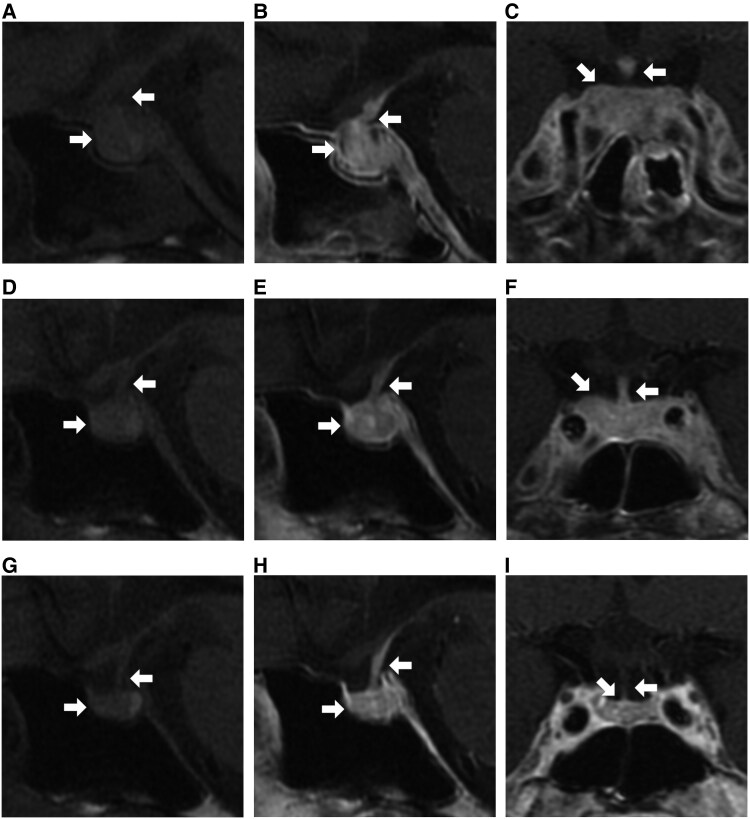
Serial pituitary magnetic resonance imaging (MRI). Noncontrast-enhanced T1-weighted sagittal images (A, D, and G), contrast-enhanced T1-weighted sagittal images (B, E, and H), and contrast-enhanced T1-weighted coronal images (C, F, and I) are shown. On admission (A–C), diffuse enlargement and homogeneous contrast enhancement of the pituitary gland and stalk (white arrow) were observed without optic chiasm compression. On day 21 (D–F), reduction in pituitary enlargement (white arrow) was noted following glucocorticoid therapy. At 5 months after discharge (G–I), further improvement with near-complete resolution of pituitary enlargement (white arrow) was observed.
